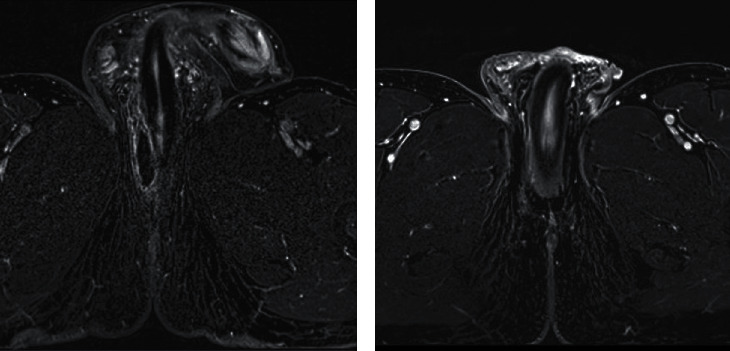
T1-weighted venous time axial pelvis MRI; (a) 8 mm fracture of the corpus cavernosum; (b) complete resolution at six weeks.
